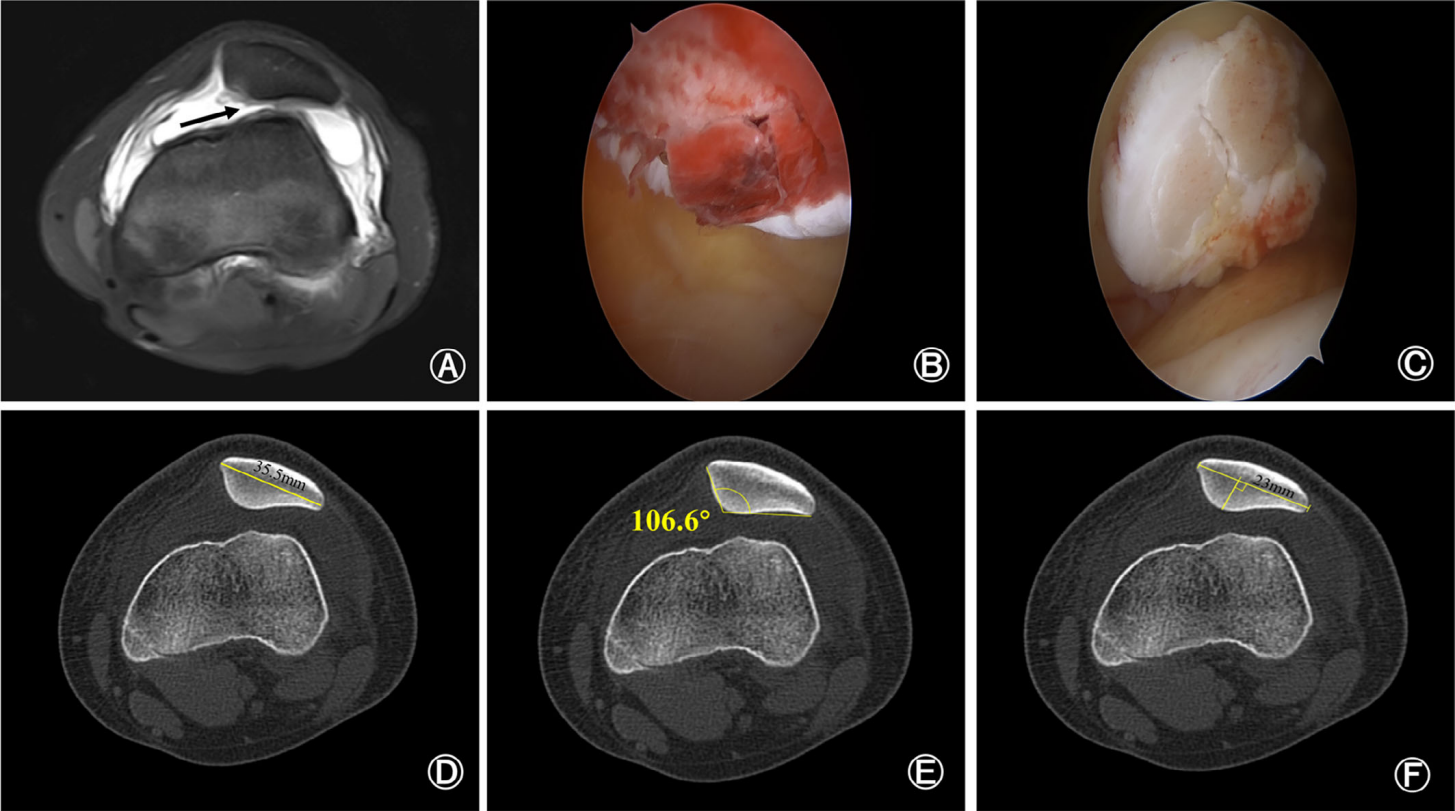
Preoperative magnetic resonance imaging and intraoperative arthroscopic findings of a 14‐year‐old patellar dislocation patient with osteochondral fracture. (A) Preoperative magnetic resonance imaging showed the fracture site on patella (black arrow). (B, C) The fracture site (B) and the fracture block covering the cartilage (C) was found under arthroscopy. (D–F) Preoperative computed tomography showed that the width of patella was 35.5 millimeters (D), Wiberg angle was 106.6 degrees (E) and Wiberg index was 0.65 (F).
